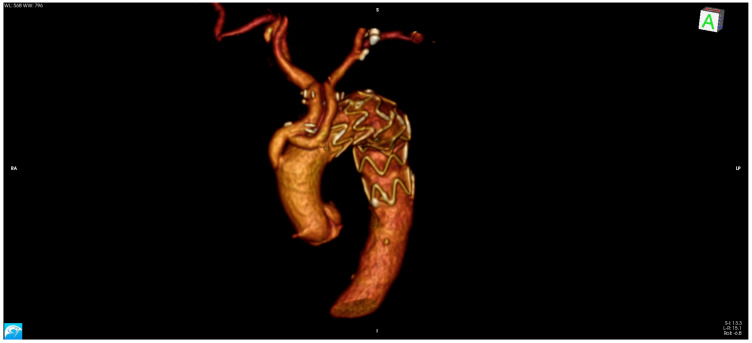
Three-dimensional (3D) angio-CT reconstruction of Zone 0 Type I Hybrid repair.
